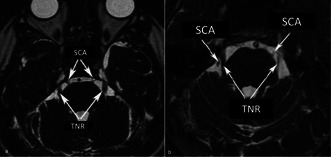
FIGURE 1 Magnetic resonance imaging: a) there is no conflict between the trigeminal nerve root (TNR) and the superior cerebellar artery (SCA); b) neurovascular conflict between the trigeminal nerve root andthe superior cerebellar nerve on the left.
